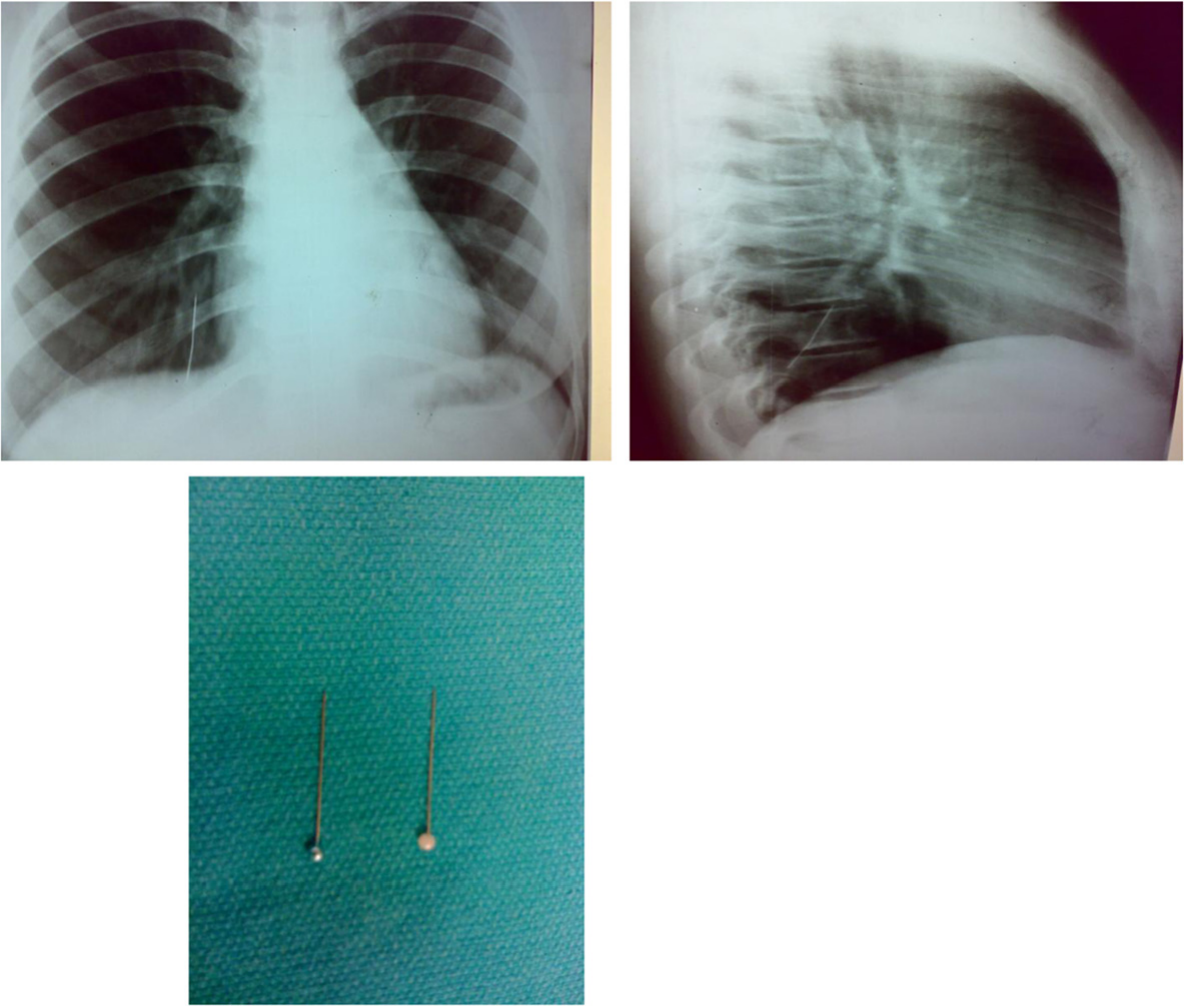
Posterio-anterior (left) and laterl (right) X-ray of a patients who inhaled a metallic hair pin (bottom).
